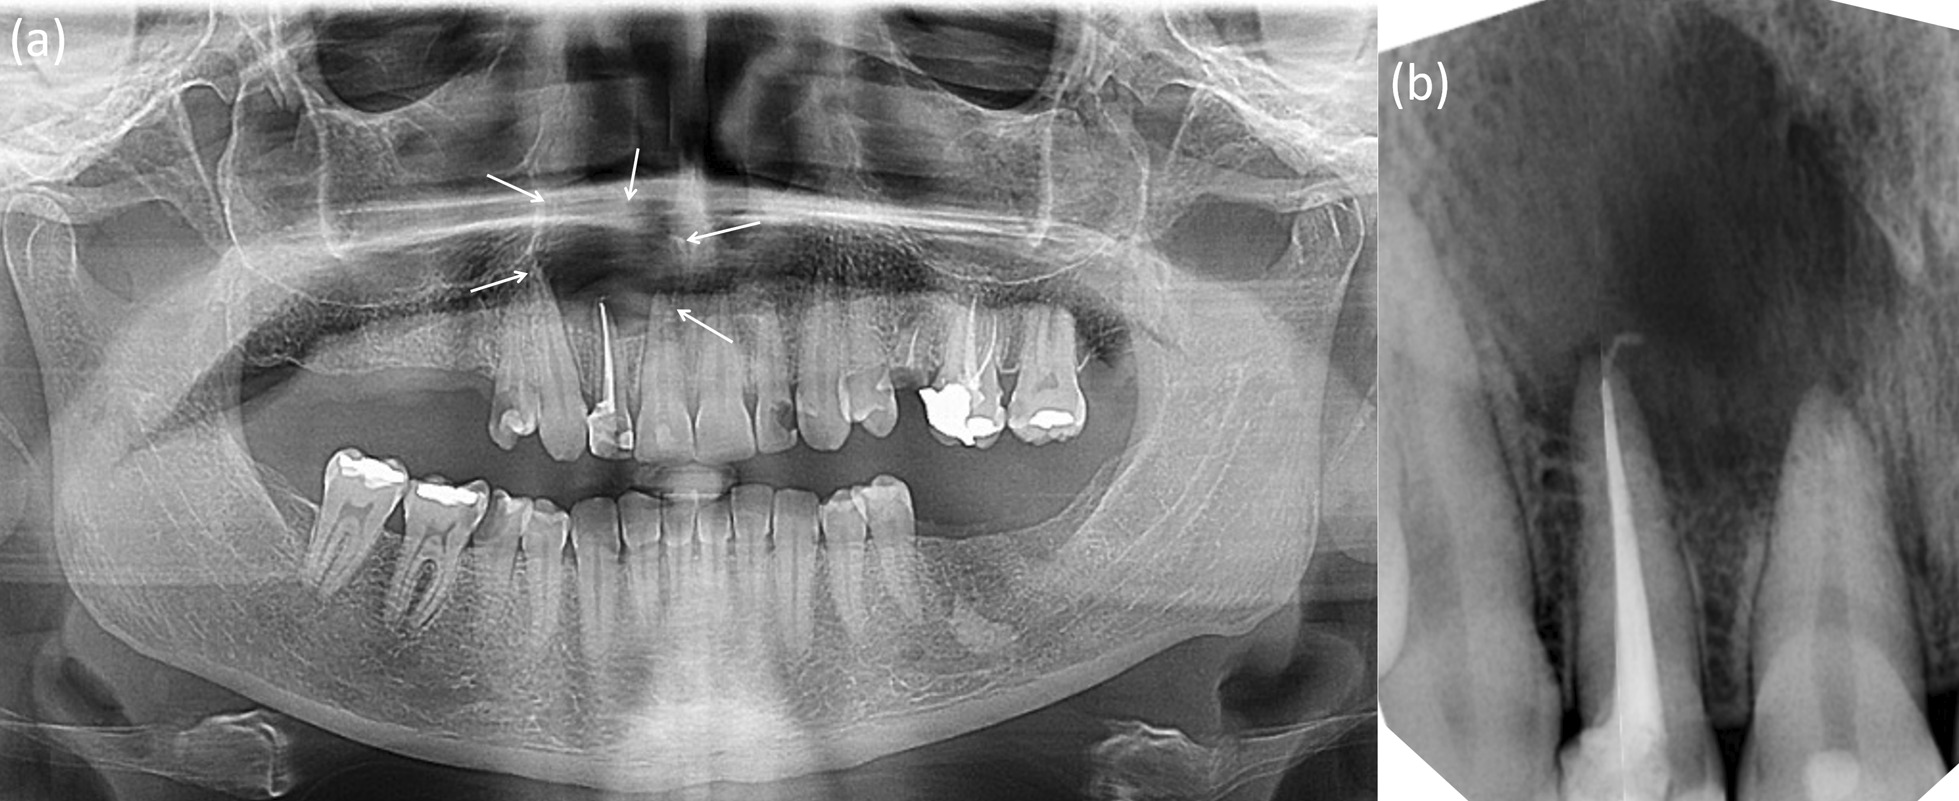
Radiographic x-ray taken after root canal treatment on tooth 12. a Panoramic radiograph showing radiolucency extending from tooth 13 to 11 (arrows) b Periapical radiograph. Note the normal pulpal morphologies of teeth 13 and 11
